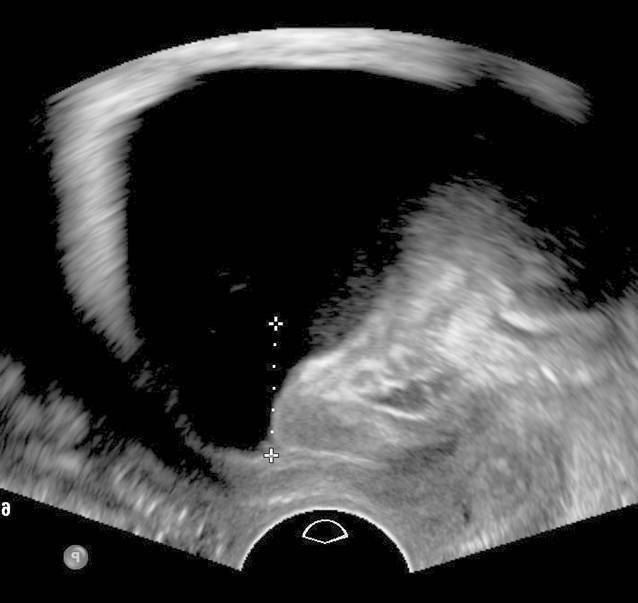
Die Beurteilung des periurethralen Gewebes in 2 Ebenen, ggf. auch mittels Farbdoppler, ist zum Nachweis bzw. Ausschluss von Pathologien, wie z. B. periurethralen Raumforderungen (Tumoren, Zysten, Abszesse, Varikosis oder Divertikel) geeignet.
